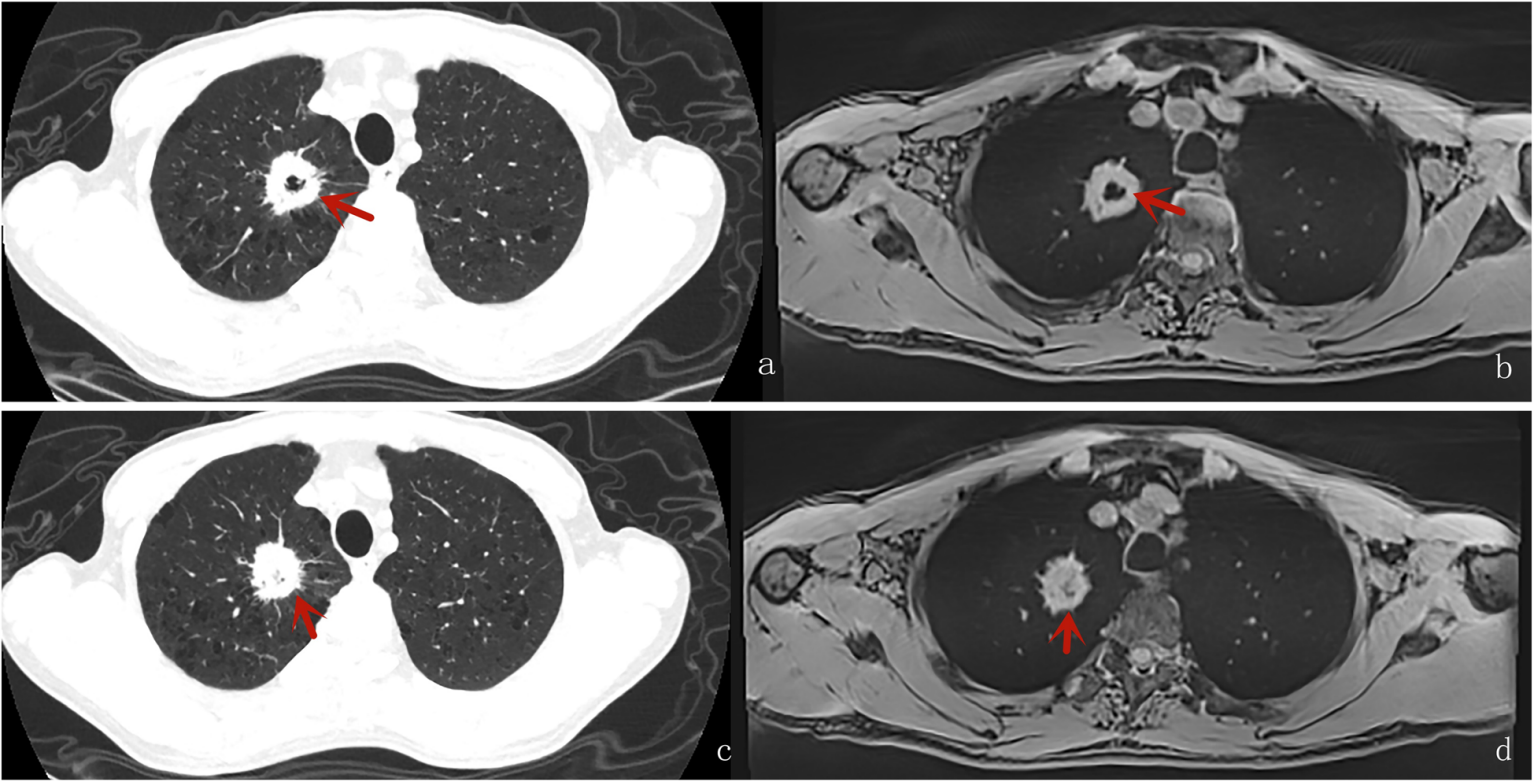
A male with adenocarcinoma; axial CT (A) and MR (C) showed cavitation (the red arrow); and sign of Spiculation was visible (the red arrow) in axial CT(C) and MR (D)
